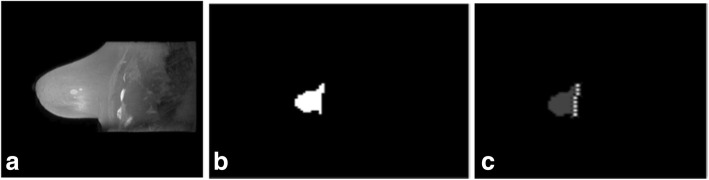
Segmentation of the nipple and final mask of the nipple-areola complex. a MIP image (maximum intensity projection) performed on T2-weighted image and seed selection. b Segmentation of the nipple (region growing technique) and final mask of the nipple areola complex. c Interpolation of points on nipple base
